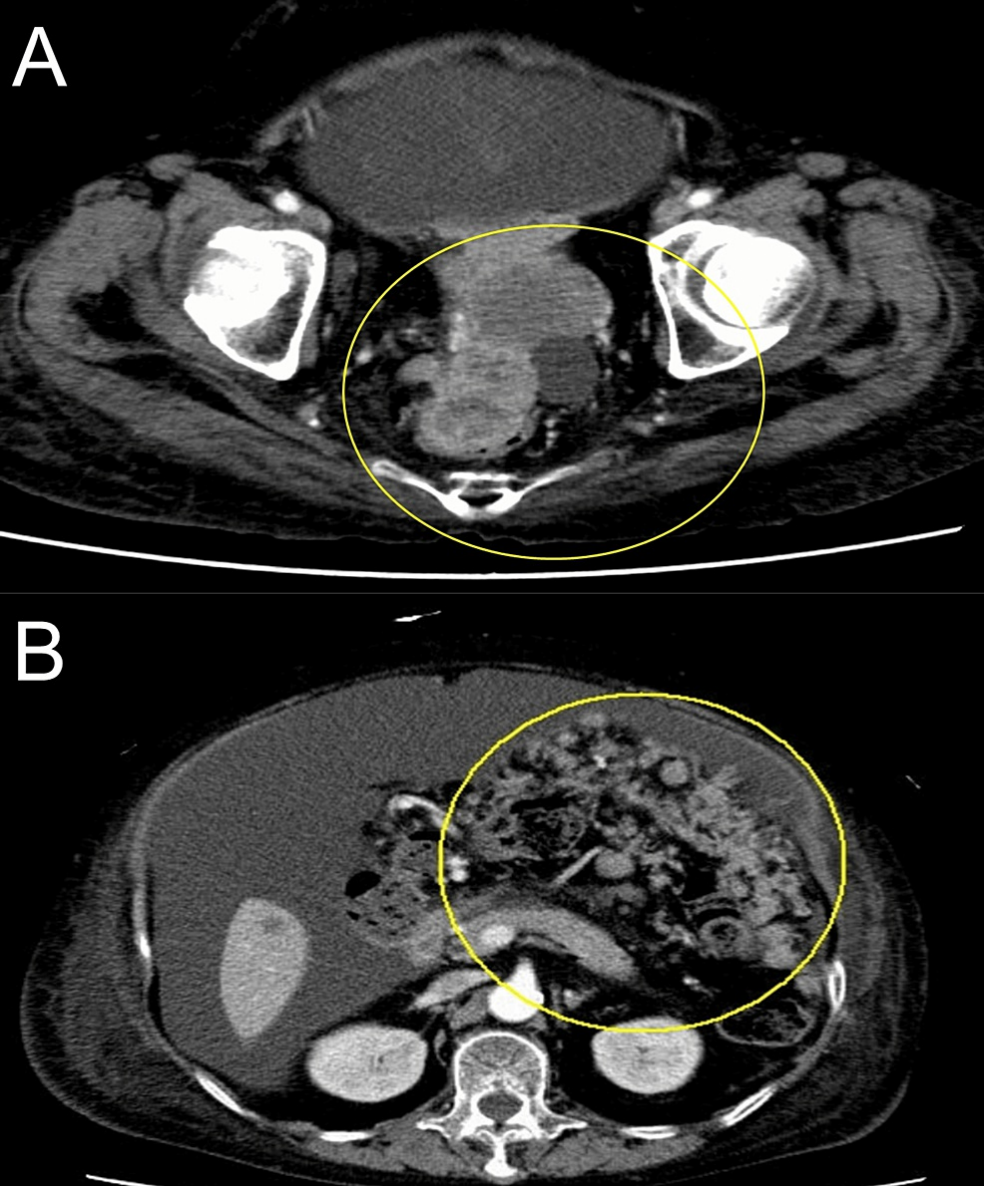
Computed tomography imaging of disseminated peritoneal nodules (A) and the large tumor (B)
